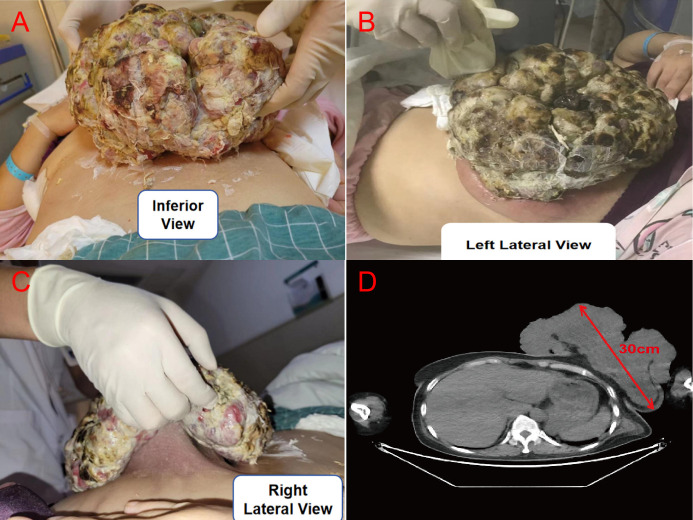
Clinical presentation and imaging of the giant pleomorphic undifferentiated sarcoma of the breast at admission. (A) Inferior view (B) Left lateral view (C) Right lateral view (D) Axial contrast-enhanced CT.
